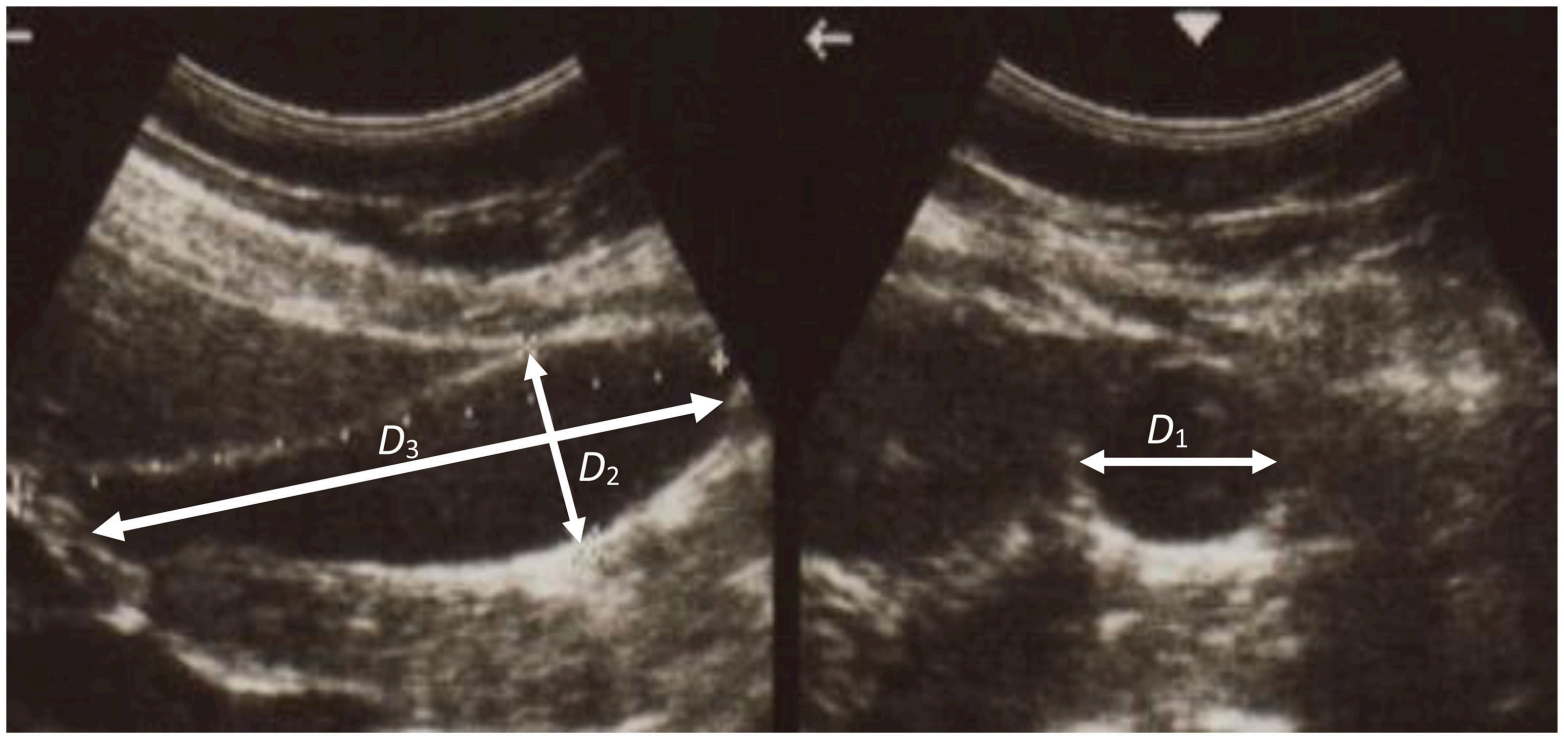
A typical ultrasonic image of human gallbladder during the emptying phase.
